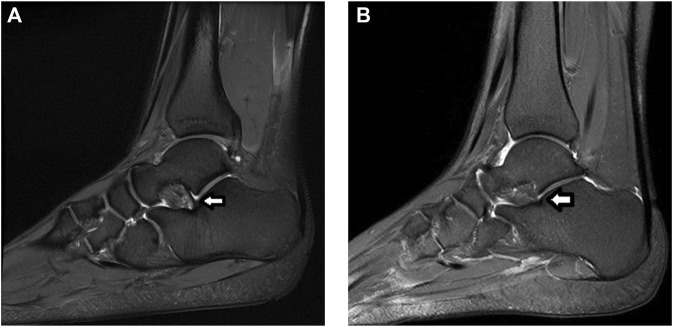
MRI assessment of the ACaL (white arrow) on sagittal image. Proton density image with fat suppression. (A). Normal ACaL. (B). Abnormal ACaL.
